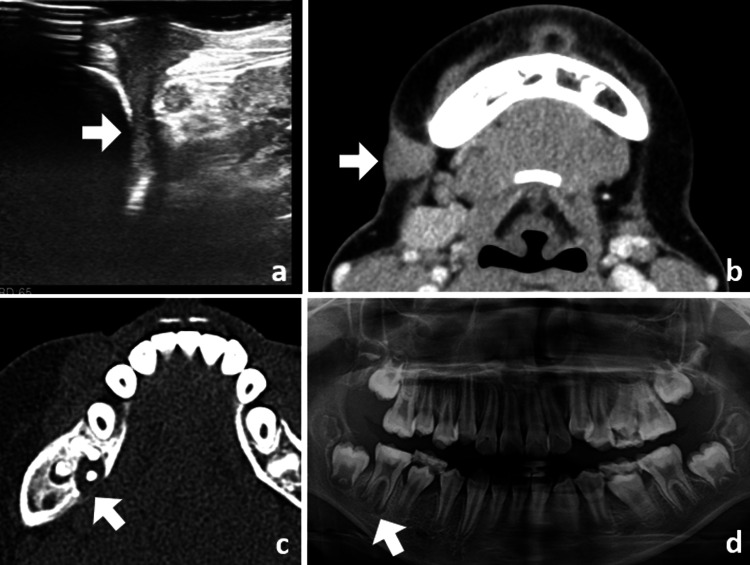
Preoperative images (a) Ultrasonography showing a fistula extending from the skin to the mandible. (b) An axial CT scan showing fistula formation and associated inflammation. (c) A focal cortical bone defect in the mandibular right first molar, and interruption of the lingual cortex and periosteal reaction. (d) A panoramic radiograph showing an enlarged periodontal ligament and apical periodontitis in the mandibular right first molar, with osteosclerosis around it. (All indicated by arrows)
